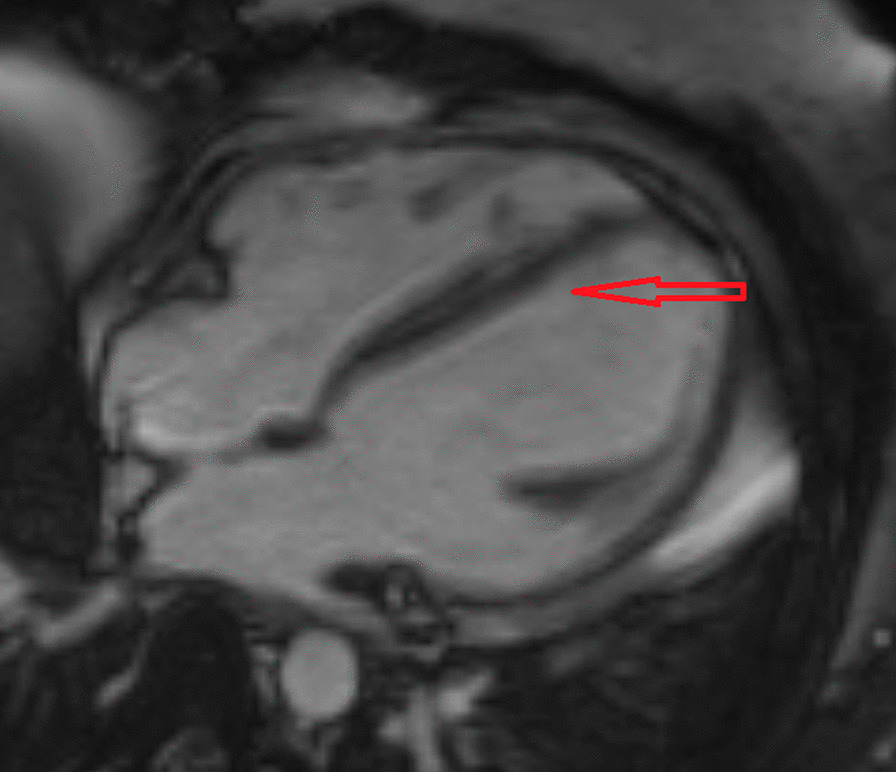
Cardiac magnetic resonance image in four chamber view showing subepicardial late gadolinium enhancement in the interventricular septum suggesting previous myocarditis
